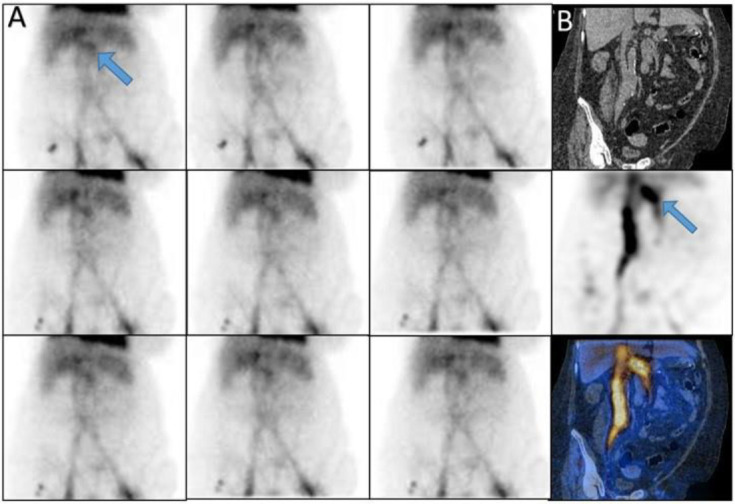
Portal vein on planar (A) and SPECT/CT (B)
